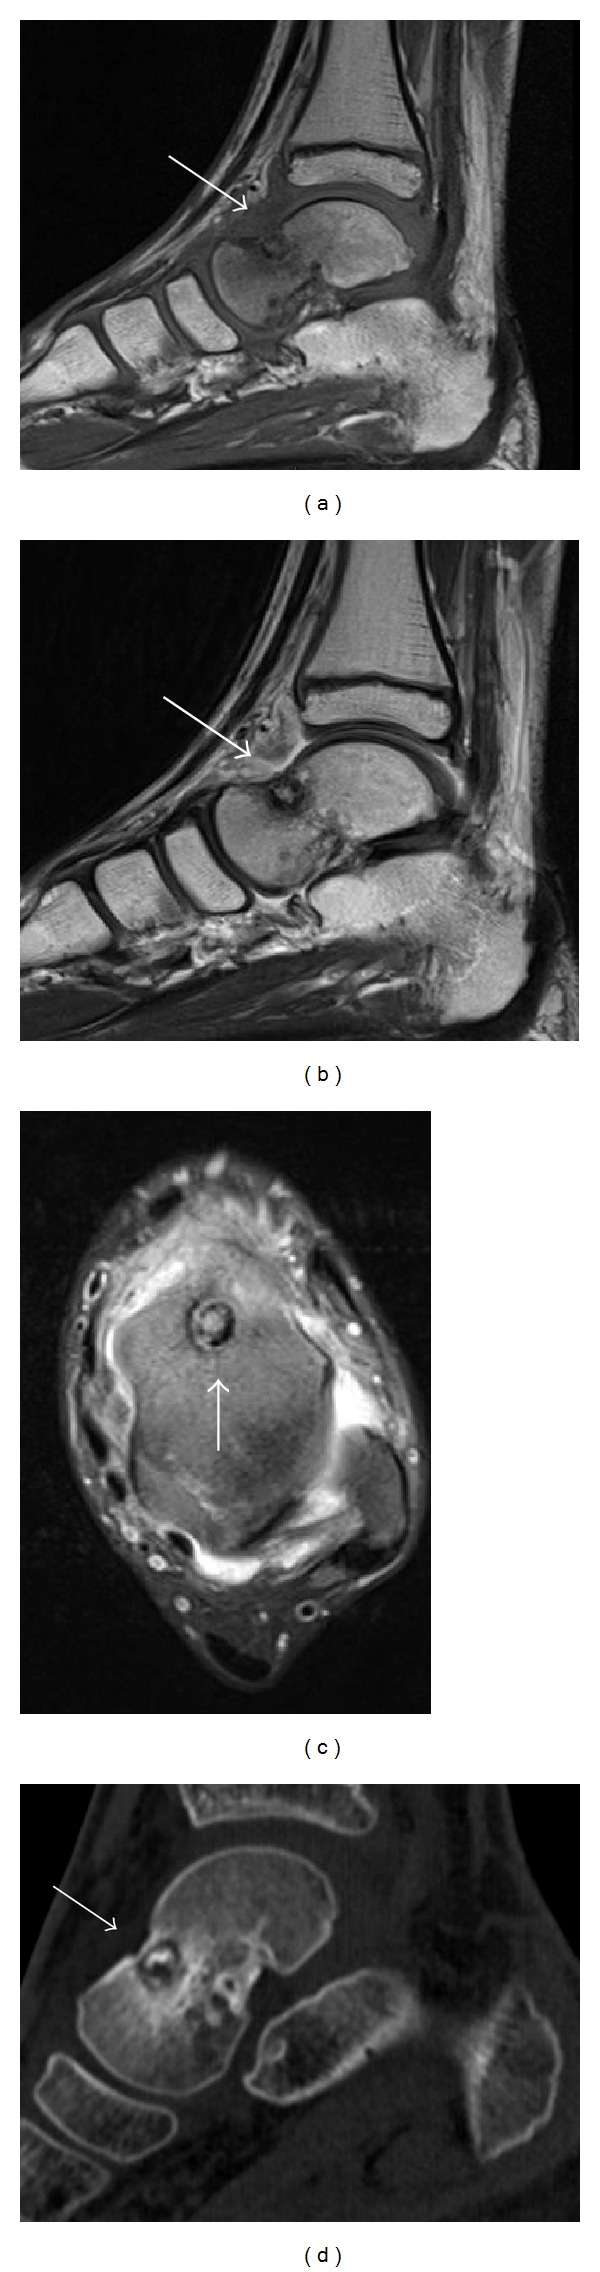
Same patient as in Figure 3. (a–c) MRI of the left ankle demonstrates the talar neck nidus, surrounded by abundant oedema and synovial inflammation ((a) sagittal T1-WI, (b) sagittal T2-WI, and (c) axial fat saturated PD-WI). (d) CT-scan of the left ankle also demonstrates the talar neck nidus.
